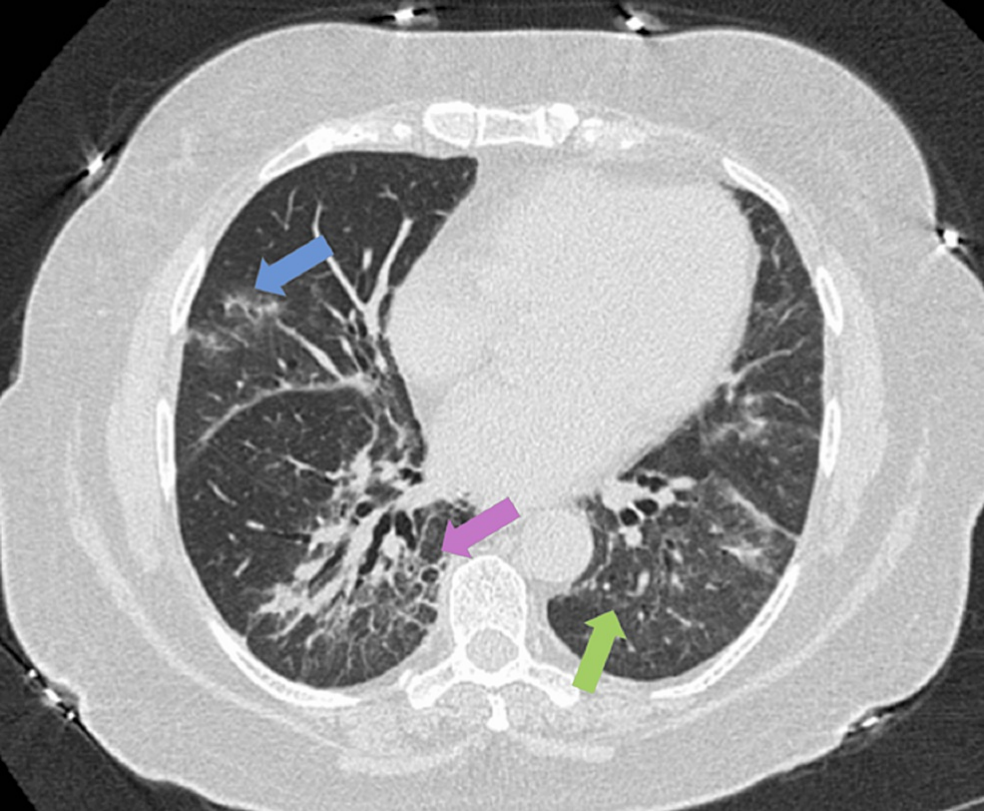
HRCT after two rounds of mepolizumab High-resolution CT thorax (HRCT) after two rounds of mepolizumab administration. Ground glass opacities (blue arrow) and reticular opacities (green arrow) appear mildly improved compared to the previous; however, bronchiectatic changes were noted in lower lobes (purple arrow).
